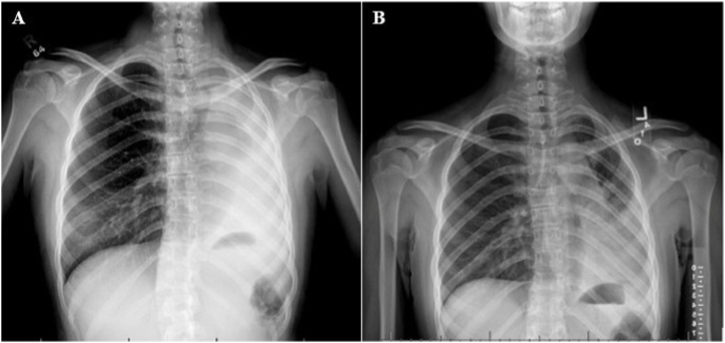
X-rays for case 2. A) Chest x-ray obtained at the time of presentation showed left hemithorax opacification with left-sided mediastinal shift. B) A spine x-ray to evaluate for scoliosis obtained one-year prior to her episode of large-volume hemoptysis showed airspace disease in the left lower lobe and lingula.
